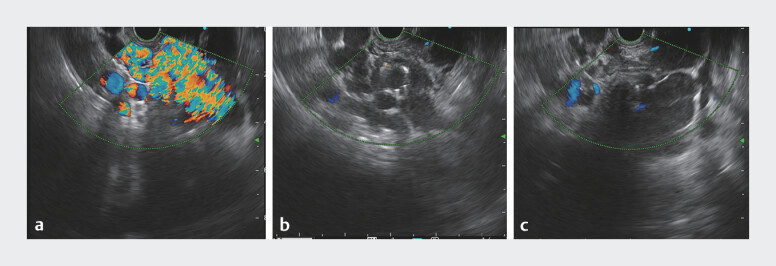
Color Doppler flow imaging.
